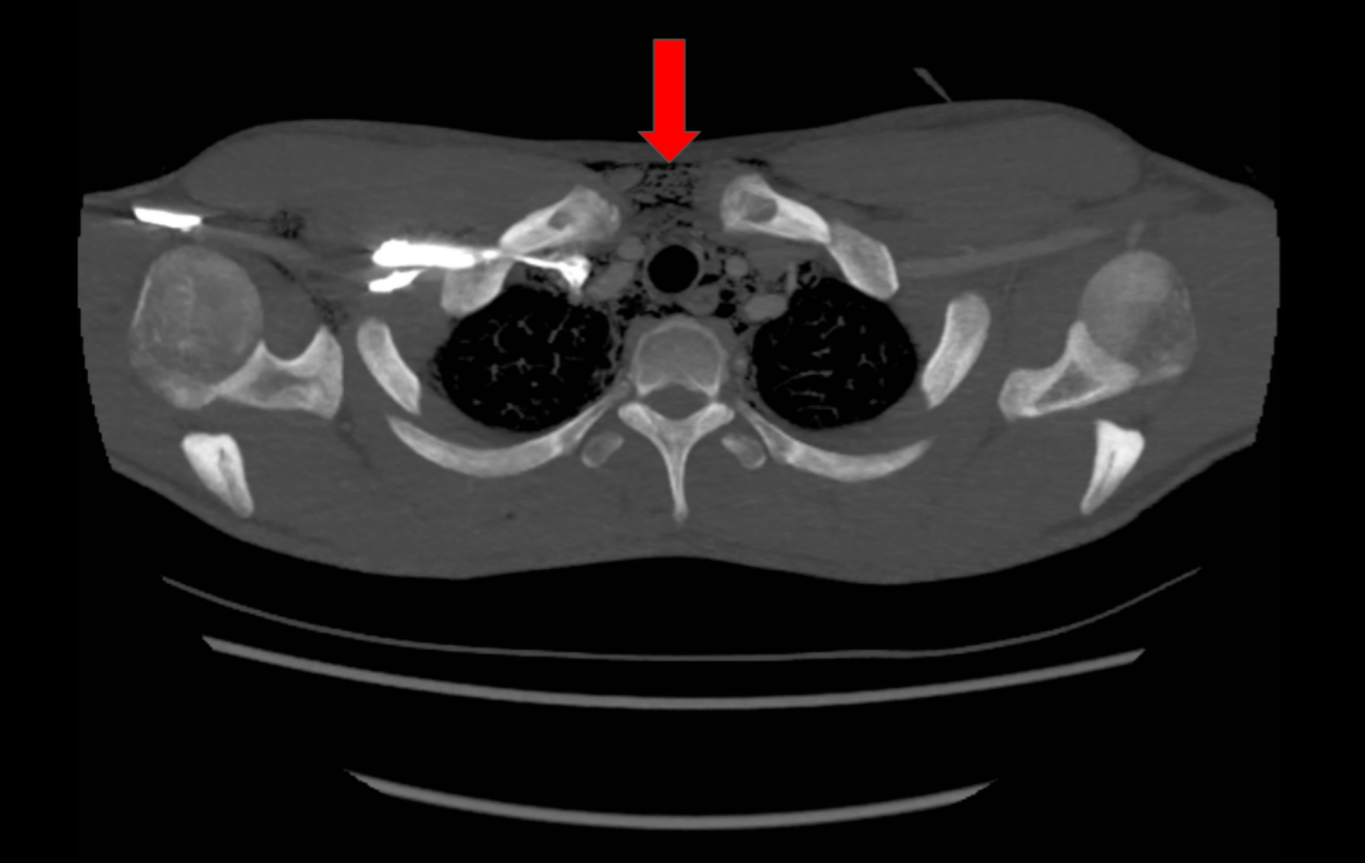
Transverse computed tomography (CT) showing air in the upper mediastinum and soft tissues of the thorax (red arrow)
